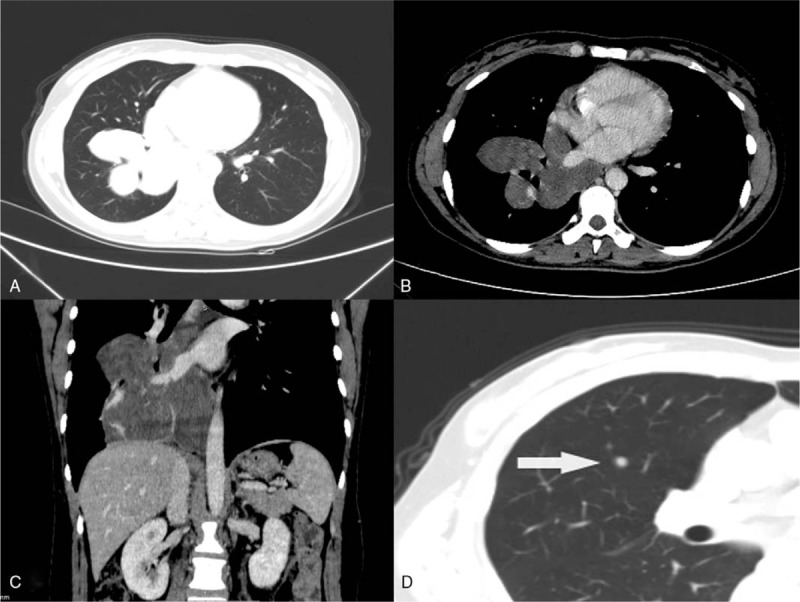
A 36-year-old woman with pulmonary benign metastasizing leiomyoma. (A, B, and C) Axial lung window, enhanced axial mediastinal window and enhanced coronal mediastinal window of computed tomography images showed a large tumor (Revise:13.2 cm × 11.1 cm × 8.9 cm) in the right lower lobe and mediastinum. (D) Axial mediastinum window of the computed tomography scan showing solid nodules (0.8 cm × 0.6 cm) in the right middle lobe (white arrows).
